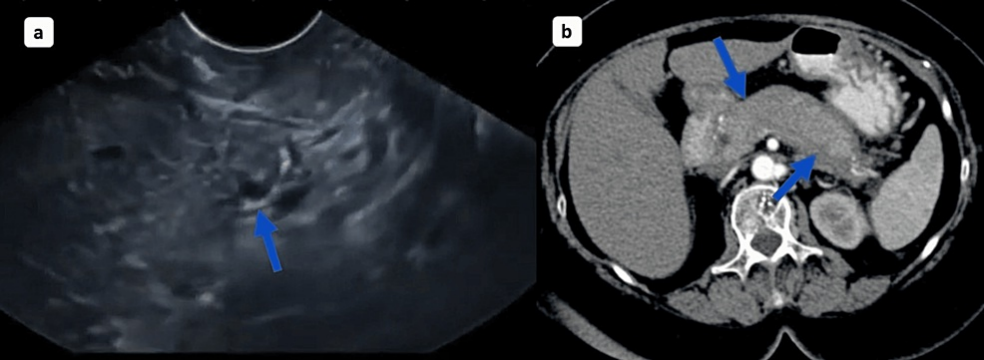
Abdominal ultrasonography reveals a dilated pancreatic duct wall (a), and CT abdomen reveals pancreatic enlargement with pancreatic head mass (b). CT: computed tomography.
